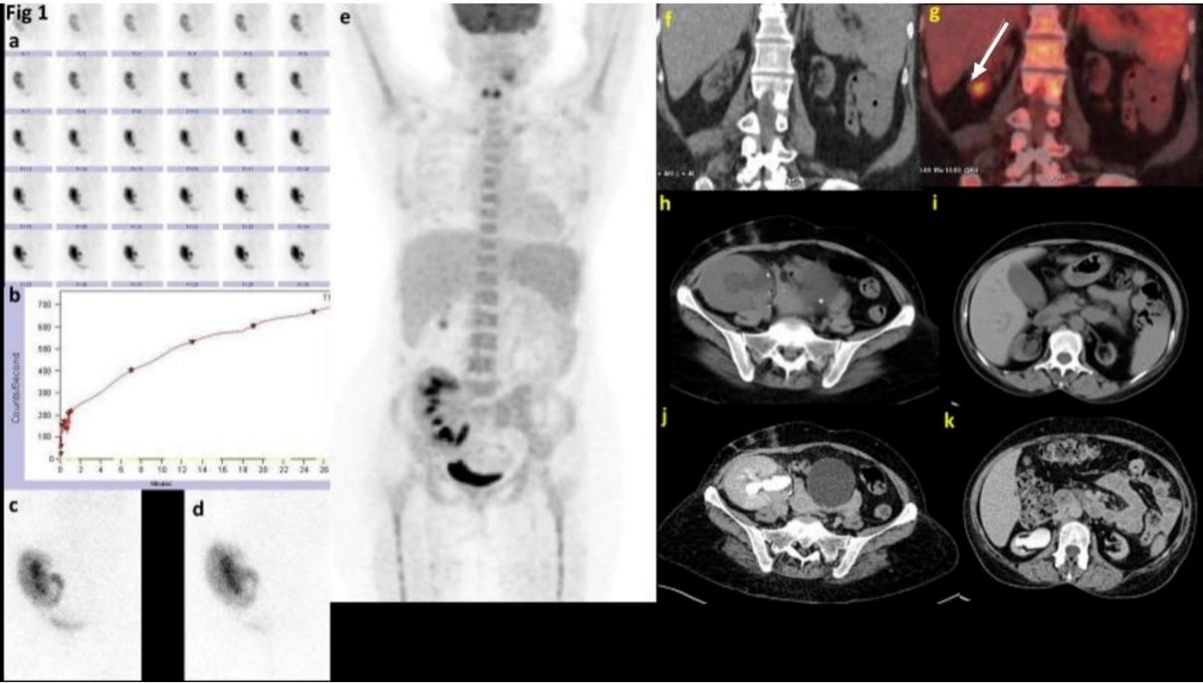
A 54-year-old man post-transplantation developing hydronephrosis in sonography (Renal scan using Tc-99m-ethylene dicysteine shows satisfactory radiotracer uptake that did not clear after Lasix). Renogram pattern was rising [a, b]. Post-void and delayed images showed significant radiotracer retention suggesting obstruction. After pyeloureterostomy, there was fever for which he had F-18-FDG PET/CT scan. It showed a hypermetabolic focus in the inferior pole of the right native kidney [g]. The CT urography showed a normal excretion of contrast from the transplanted kidney [h, j]. The right native kidney showed contrast in the delayed image suggesting reflux [k]. Note that the left native kidney shows no contrast in delayed image.)
